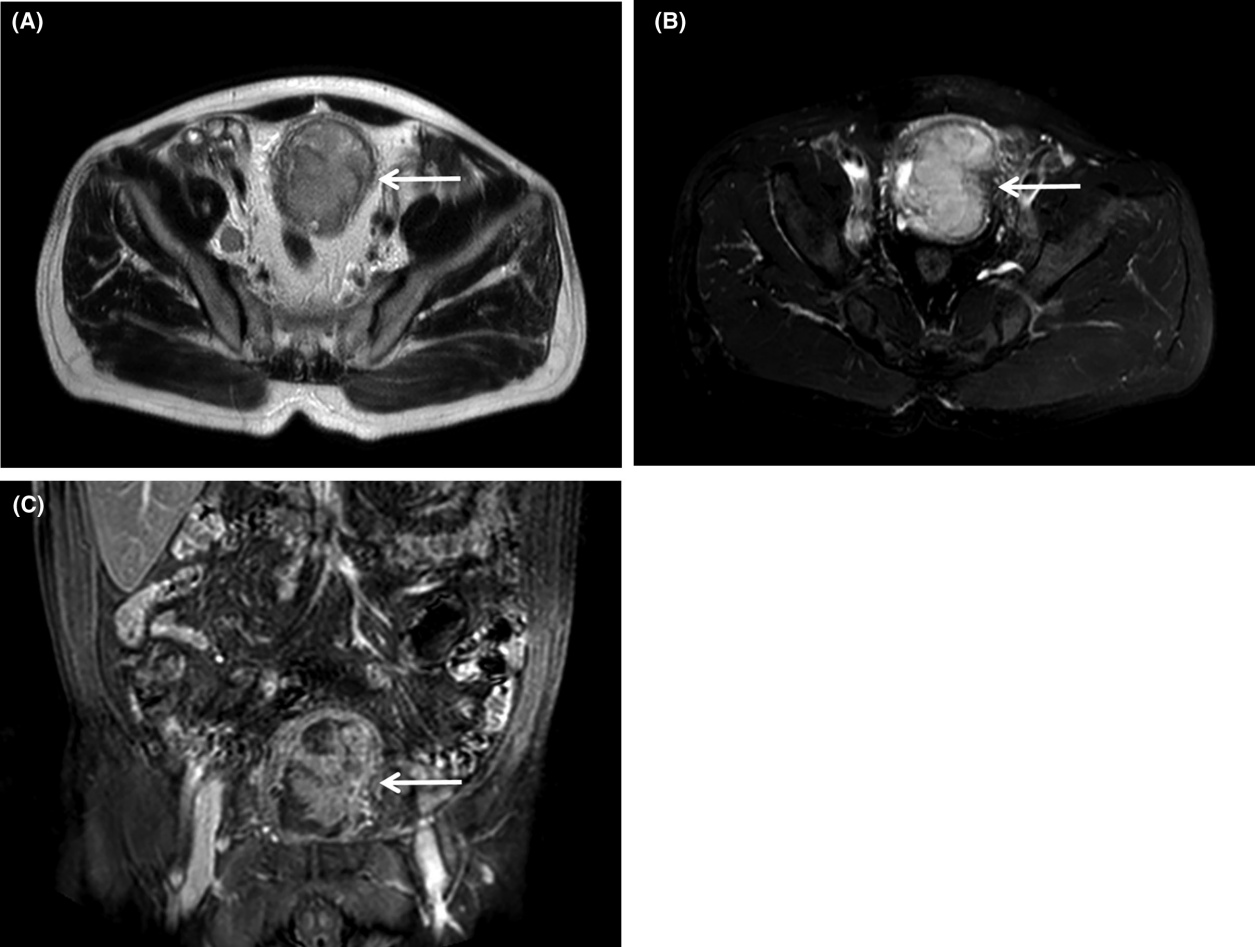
Magnetic resonance imaging of sarcomatoid carcinoma of the urinary bladder. (A) T1‐weighted imaging showed the mass (arrow) with slightly longer signals in the axial section. (B) T2‐weighted imaging showed the mass (arrow) with slightly longer signals in the axial section. (C) The enhanced scanning showed the mass (arrow) with a significant enhancement in the coronal section.
